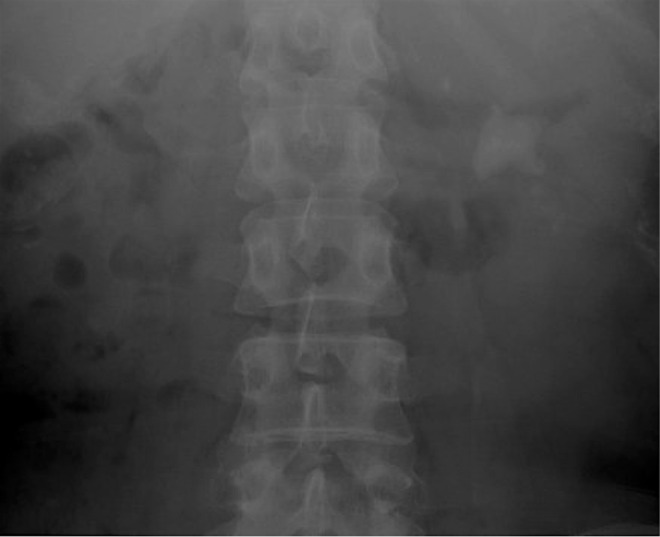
Preoperative abdominal radiograph showing large left-sided partial staghorn calculus.
